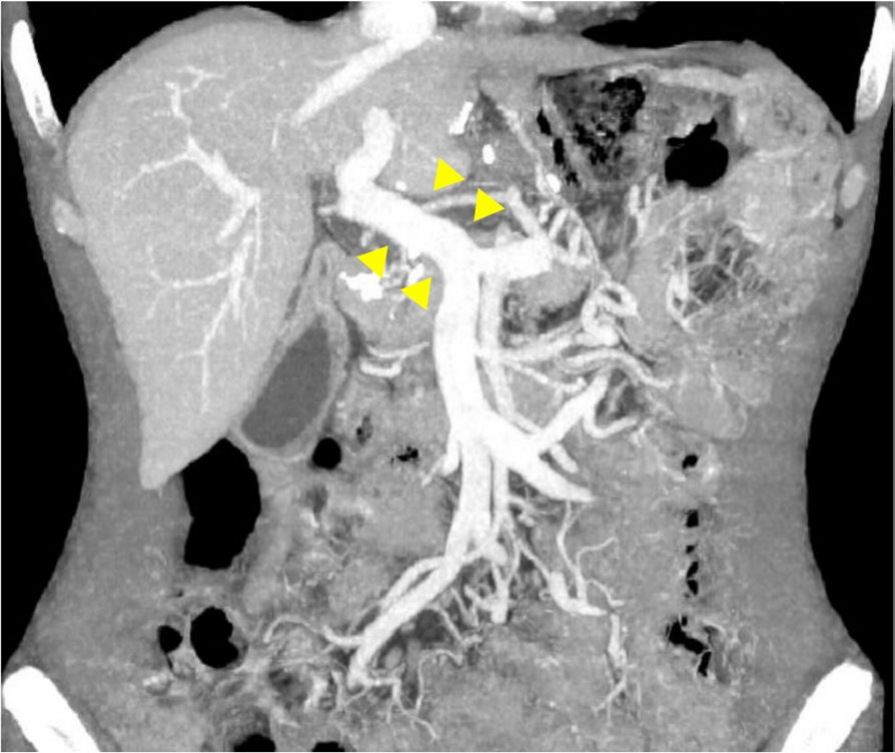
Computed tomography showed the disappearance of the portal vein tumor thrombus
